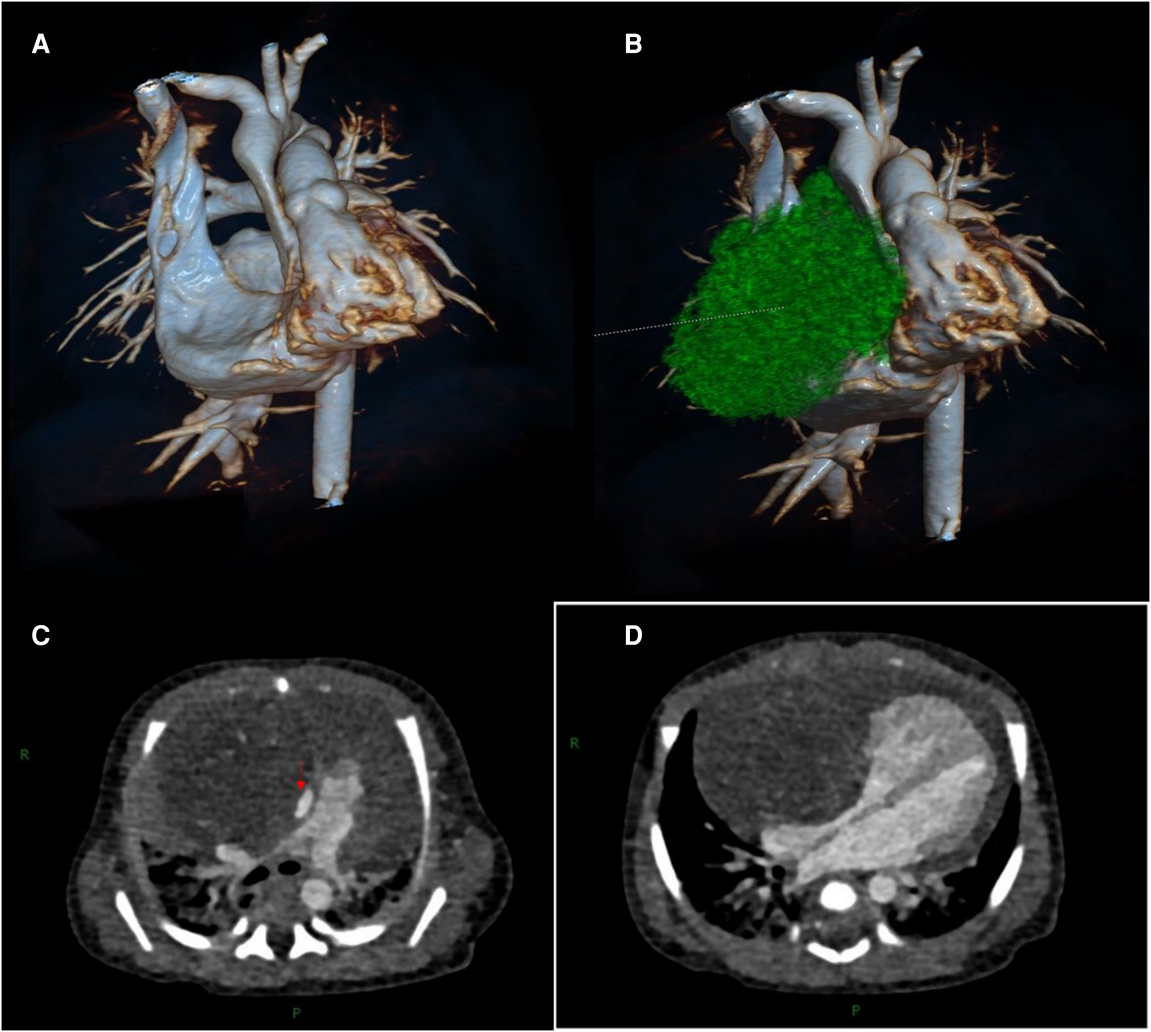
Cardiac computed tomography angiography images. (A, B) Three-dimensional modeling images show a huge mass in the right front of the heart, which severely compresses the ascending aorta and right atrium. (C) The aorta (red arrow) is compressed and flattened by the mass. (D) The mass compresses the right atrium.
